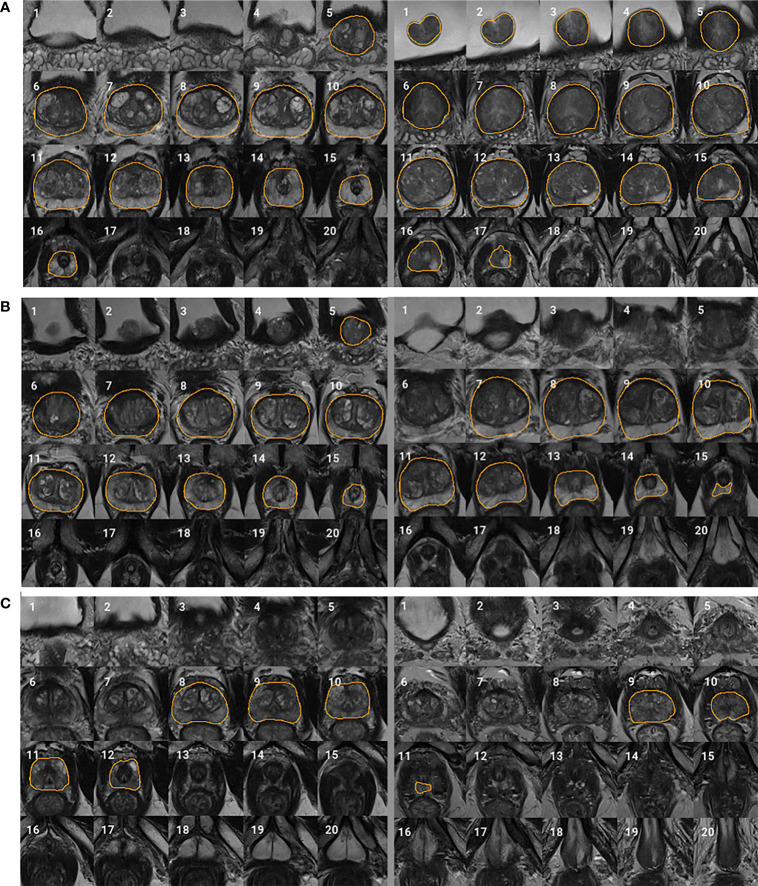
Typical examples for each visual grade. Rows (A–C) represent two segmentation examples with visual grades 3 (excellent), 2 (acceptable), and 1 (unacceptable), respectively. Slices 1–20 represent MRI slices from superior to inferior. Regions encircled by organ boundary are the prostate whole gland.
